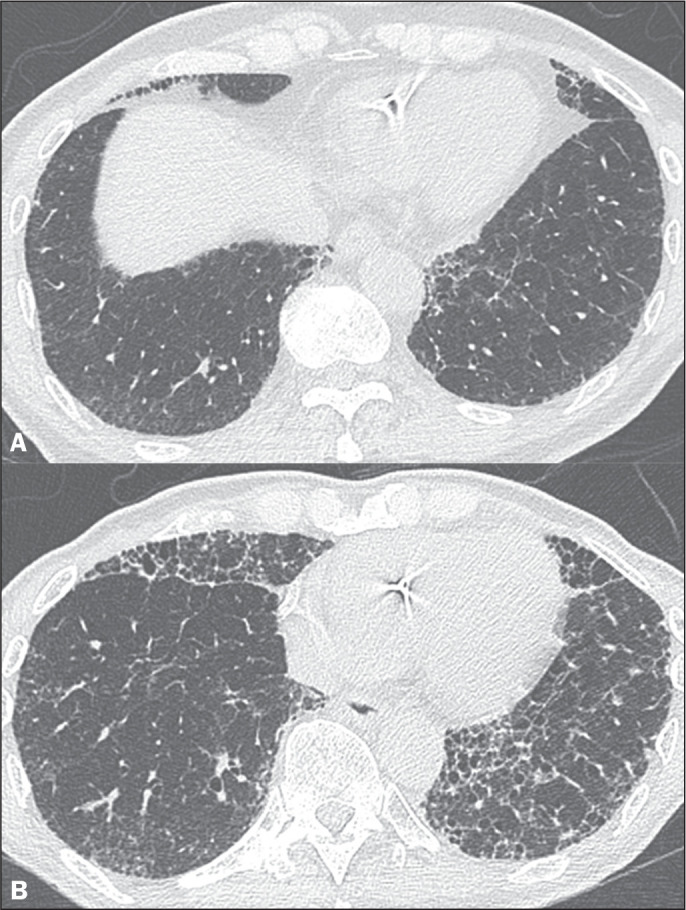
Evolution of subpleural fibrotic ILAs over five years. A: Chest CT
